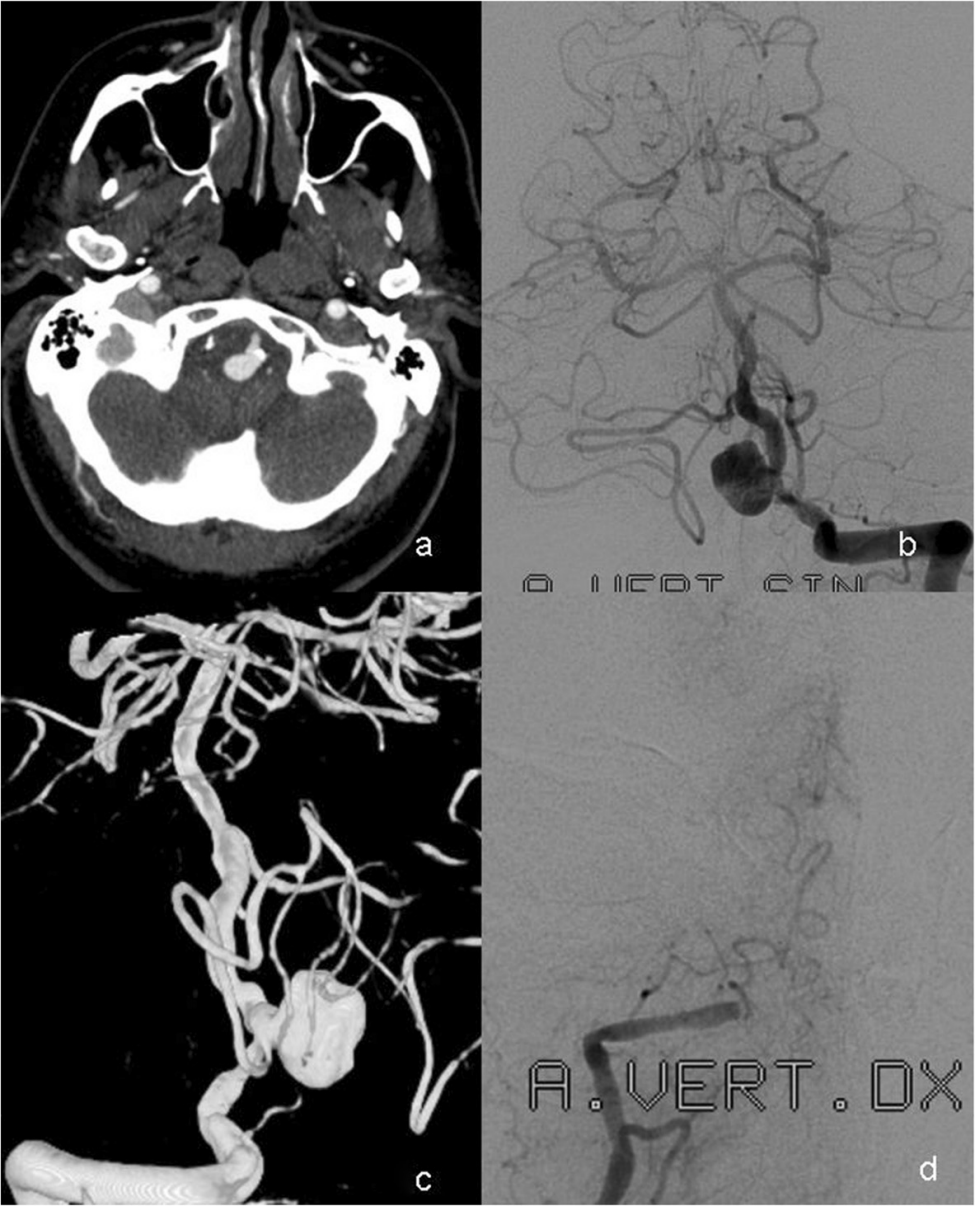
Preoperative computed tomography and angiographic findings in the posterior fossa. (a) CT angiography in a 68-year-old male showing the aneurysm with calcified neck imprinted in the brain stem. (b-c) Vertebral angiography with contrast injection in the left vertebral artery (AP projection) and rotational angiography with 3D reconstruction of the vertebro-basilar system show an irregularly shaped, saccular aneurysm localized at the left VA-PICA junction. (d) Right vertebral angiography shows distal occlusion of the V4 segment.
